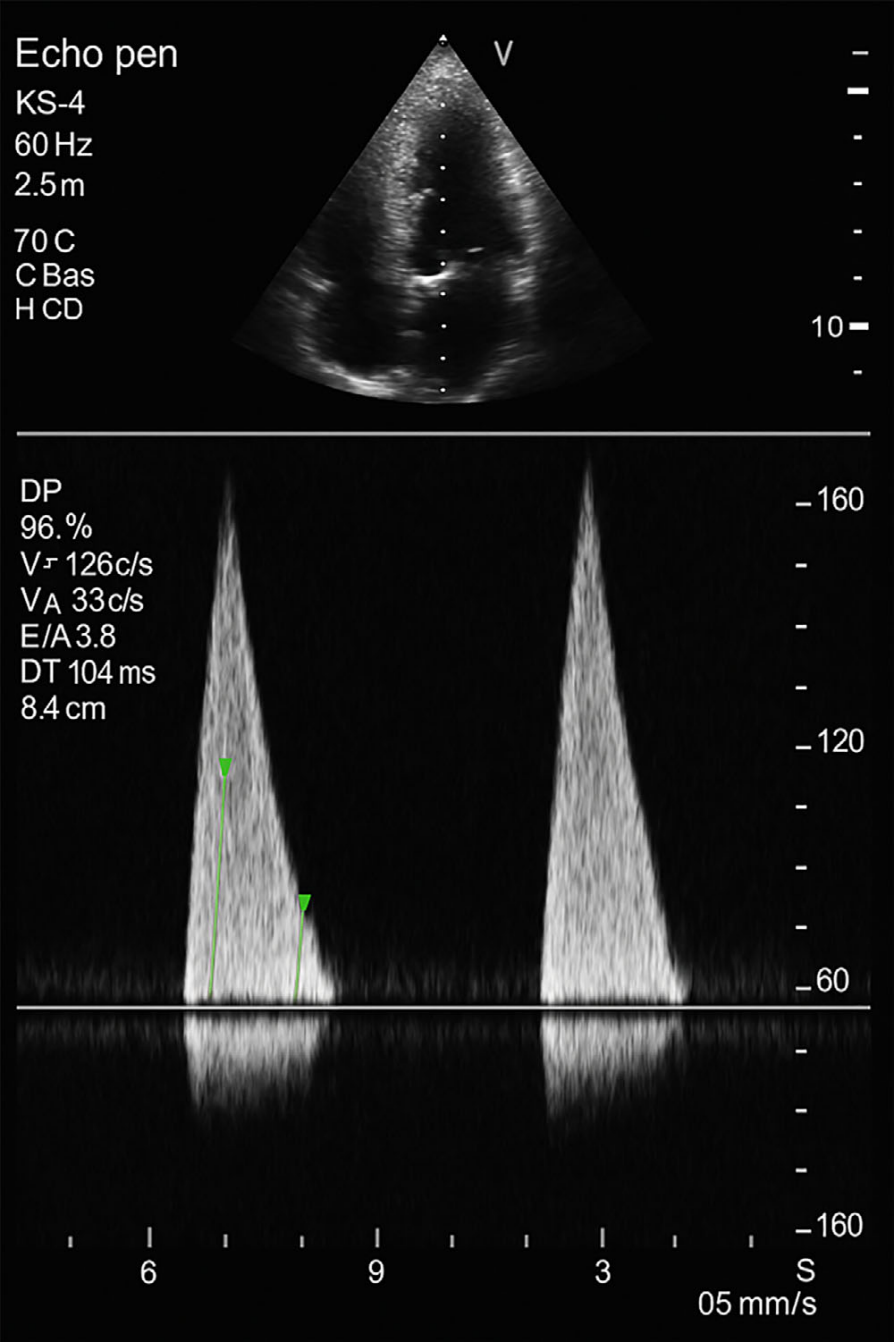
Doppler echocardiographic findings consistent with restrictive cardiomyopathy: markedly elevated early diastolic (E) velocity (126 cm/s), a diminished atrial (A) velocity (33 cm/s), an E/A ratio of 3.8, and a shortened deceleration time (DT) of 104 ms.
